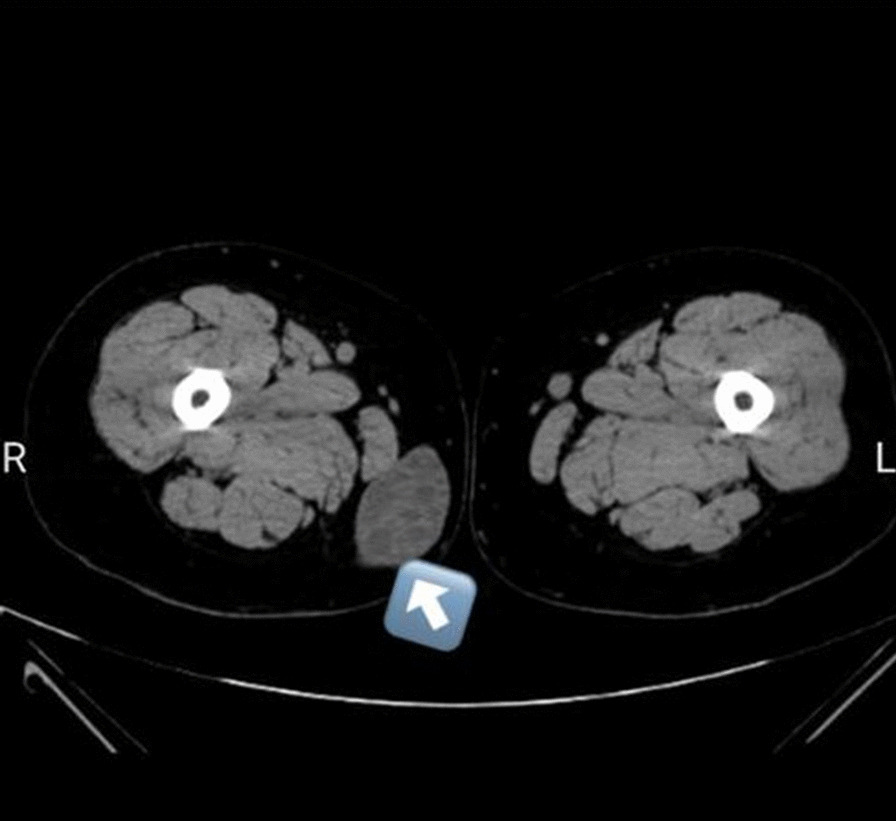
Computed tomography scan finding correlated with hydatid cysts. Multi-lobular cystic mass with no connection to the abdominal region is also observed
